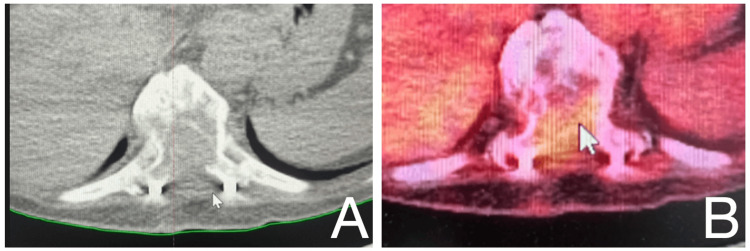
Patient 2: (A) CT scan showing destructive malignant infiltration of the thoracic vertebral body. (B) PET-CT demonstrating marked hypermetabolic activity corresponding to the same vertebral lesion, consistent with metastatic involvement. CT: computed tomography; PET-CT: positron emission tomography-computed tomography.
